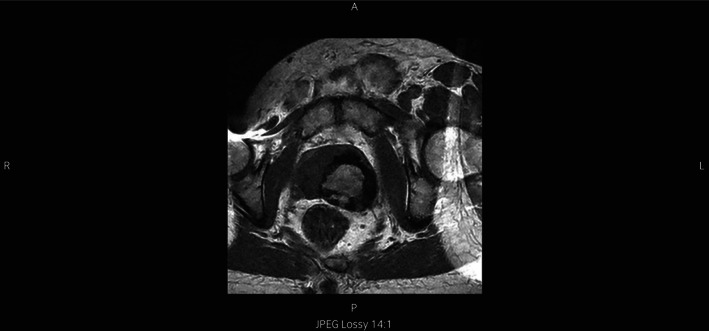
MRI of the pelvis sharply delineates a dominant mass in the left lobe of the prostate.
